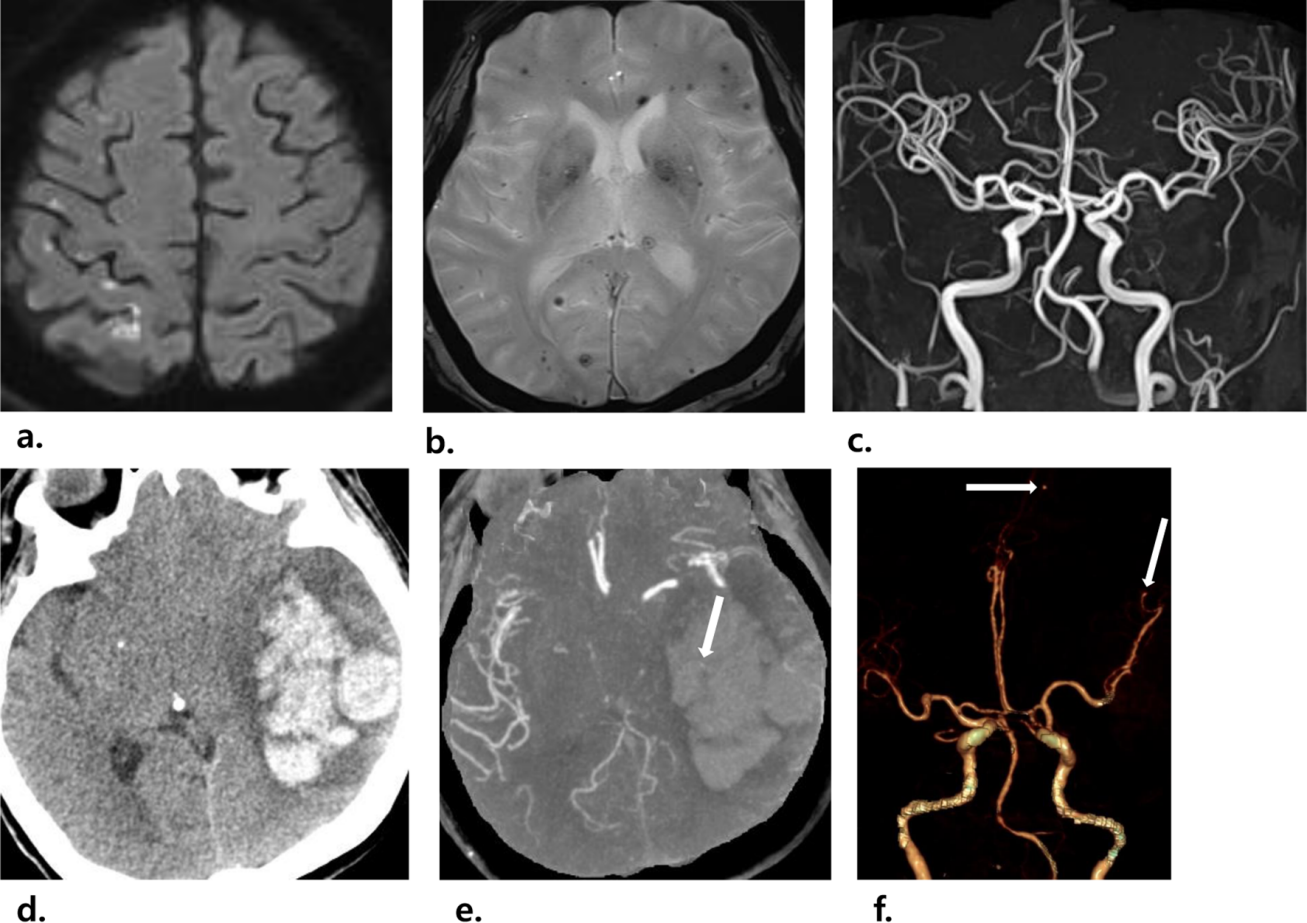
Images of a 69-year-old woman with left-sided infective endocarditis. (a,b) Brain MRI images show multifocal, small, high-signal-intensity lesions in the right frontal and parietal lobes on the diffusion-weighted image (a), which suggests embolic infarctions, as well as multiple cerebral microbleeds in both cerebral hemispheres on the T2*-weighted image (b). (c) No definite vascular abnormality was detected on brain MR angiography. After 4 days, the patient had drowsy mentality and vomiting, and a CT scan was performed. (d) Axial non-contrast-enhanced CT imaging shows a new acute intracranial hemorrhage in the left temporoparietal lobe. (e) A small enhancing lesion (arrow in e) is seen on axial maximum-intensity projection imaging, suggesting that a infectious aneurysmal rupture caused an acute intracranial hemorrhage. (f) On the 3D volume-rendered image of CT angiography, two additional infectious aneurysms were detected in the distal branch of the left ACA and left MCA (arrows in f). Despite emergency decompressive craniectomy, the patient died.
